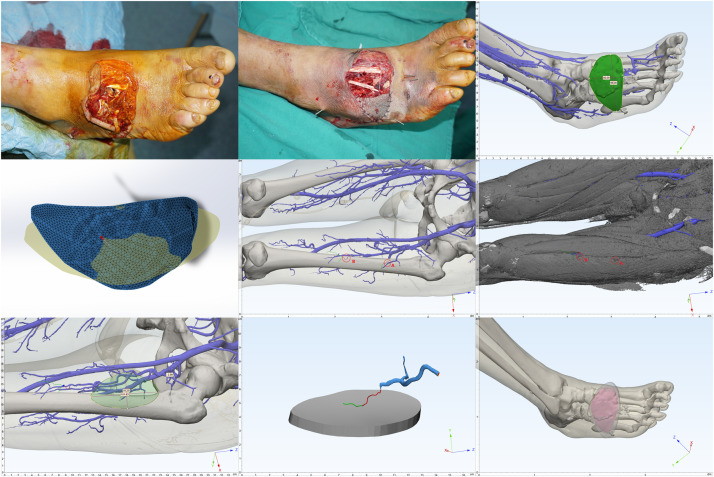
Case : A 57-year-old man who sustained a soft tissue defect on the dorsum of the right foot following a traffic accident. The defect was reconstructed with an ALTP flap. *(First line, left)* Wound appearance post-injury. *(First line, middle)* Post-debridement: 9.6 cm × 5.0 cm wound on the foot dorsum, with tendons and bones exposed. *(First line, right)* CT reconstruction of the foot, marking the right foot wound. *(Second line, left)* Flattening of the wound model. *(Second line, middle)* CTA and 3D reconstruction of the donor site, showing the location of perforators A and B. *(Second line, right)* Perforator A: musculocutaneous; Perforator B: intermuscular. *(Third line, left)* Design of the ALTP flap, harvest area 10.1 cm × 5.5 cm. *(Third line, middle)* Surgical marking outlining the planned incision for the ALTP flap harvest, based on the preoperatively identified perforators. *(Third line, right)* Intraoperative view showing the ALTP flap partially inset into the dorsal foot defect.
